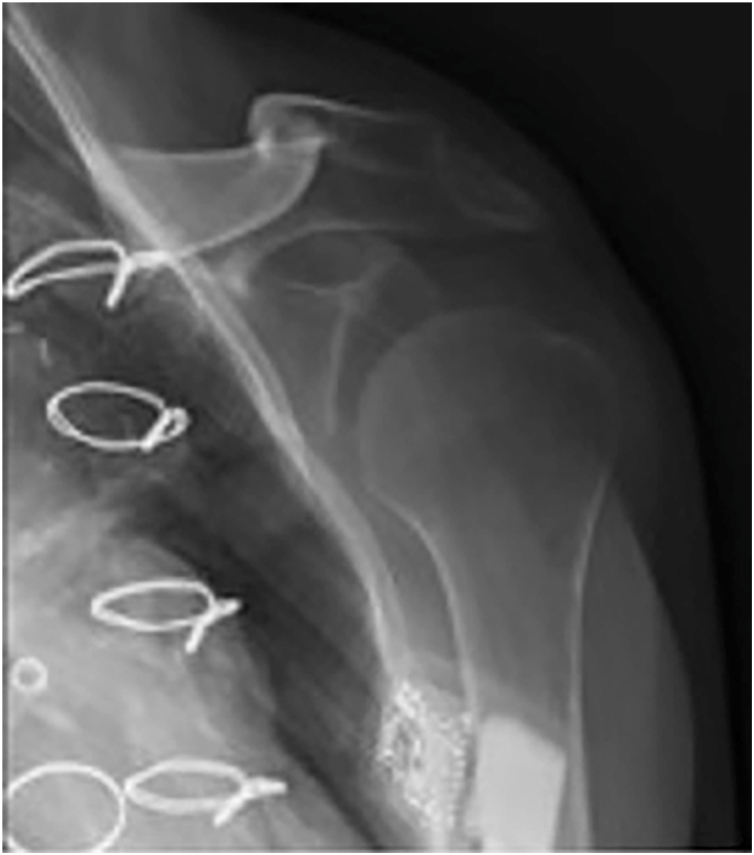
Pre-operative radiograph showing inferiorly subluxed humeral head.
